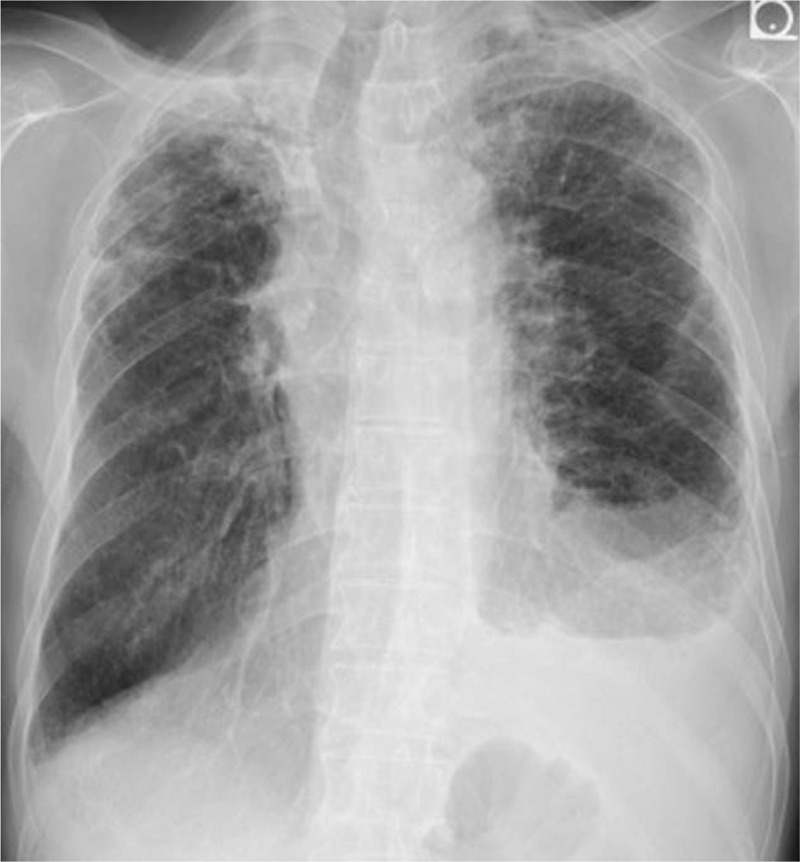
A chest radiograph showing left pleural effusion.
